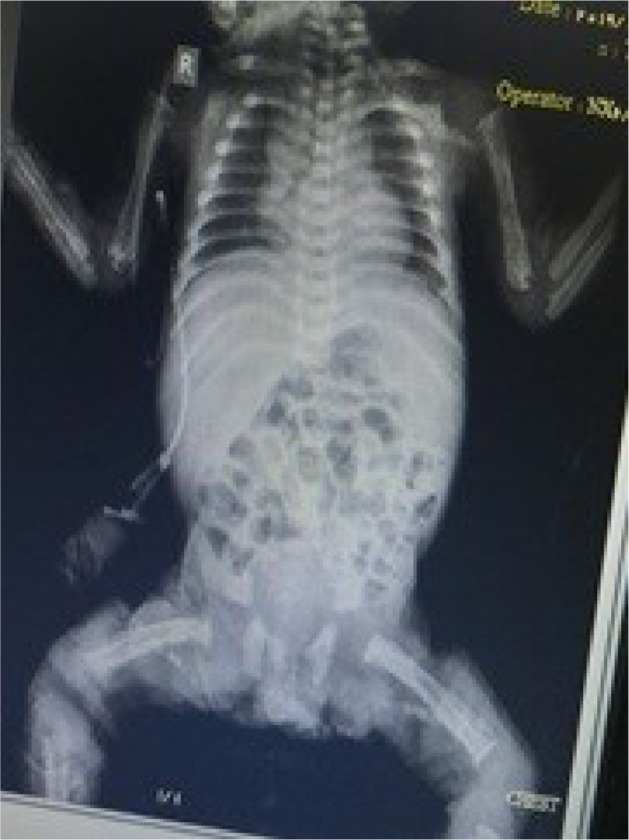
AP chest and limb X-ray.
